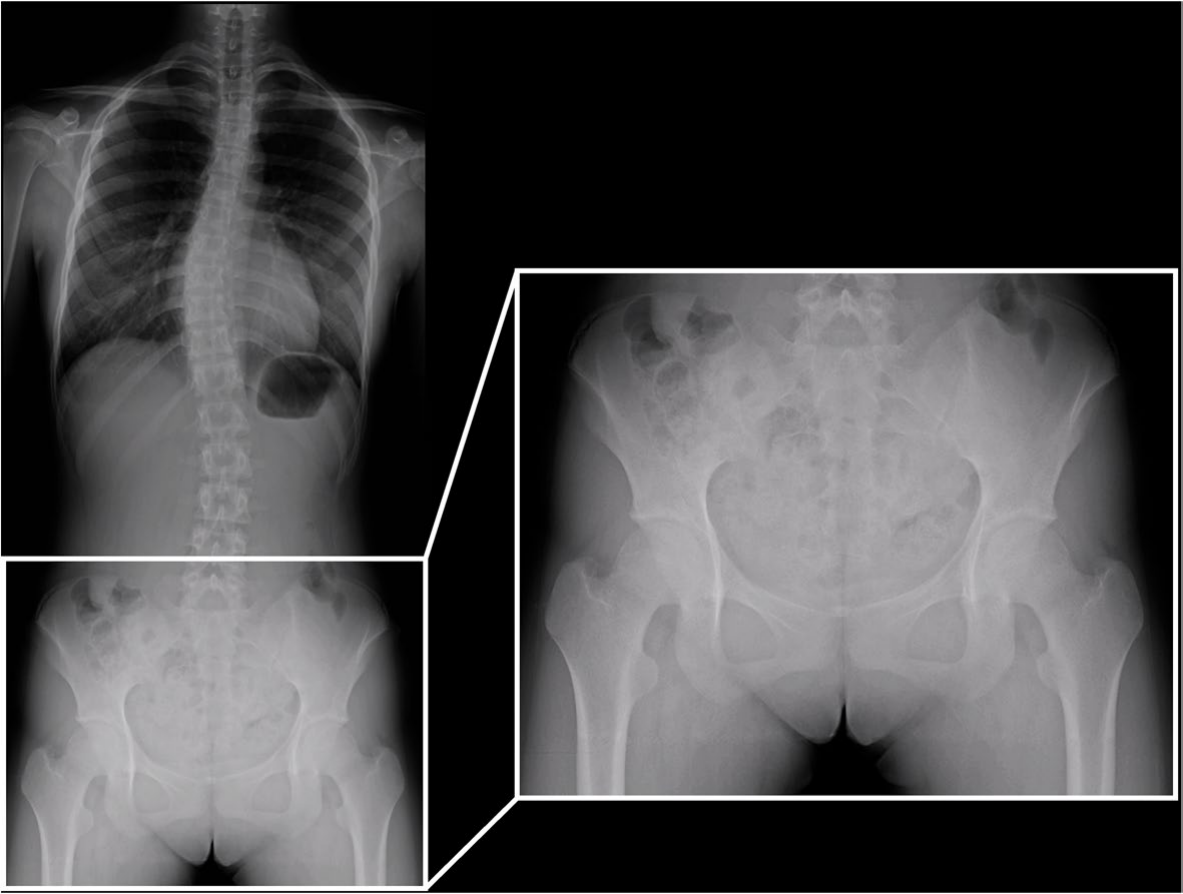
Plain standing whole-spine anteroposterior radiograph and pelvic part of radiograph. With a unified imaging method, it is possible to obtain a stable radiograph with suppressed pelvic rotation and lateral tilt. Measurement was performed using the pelvic part
